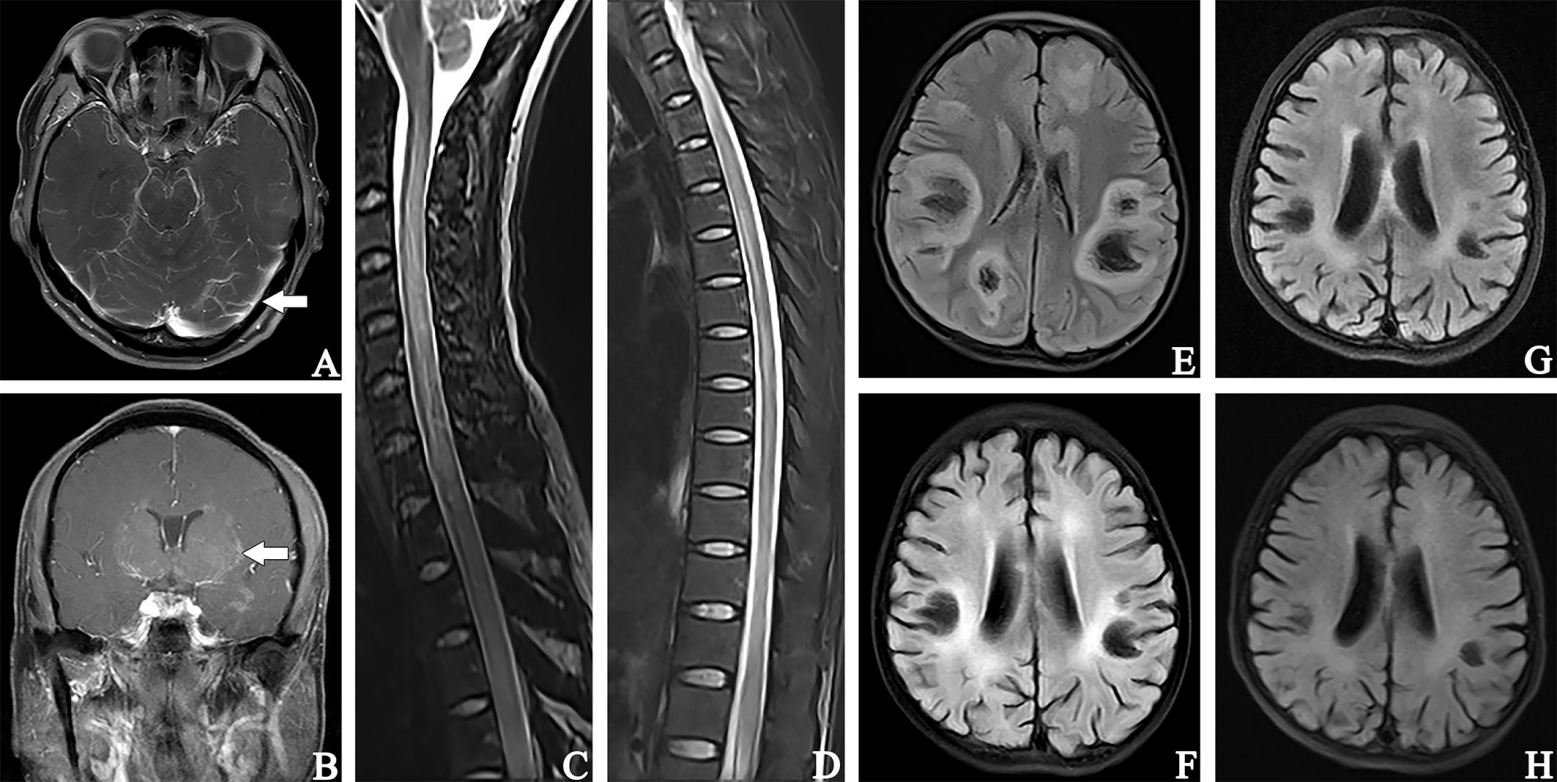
Contrast-enhanced T1-weighted magnetic resonance image (A, B). (A) patient #18, showed soft meningeal linear enhancement (arrow). (B) patient #23, MR image suggested patchy enhancement around the lateral ventricles (arrow). (C, D): patient #18, T2-hyperintense lesions in C2-T12. Fluid attenuated inversion recovery (E–H). MR images of patient #3. (E) MR image showed multiple lesions of bilateral brain parenchyma at admission. (F) (1 month after treatment with IVMP and IVIG) and (G) (2 months after treatment) showed that the extent of lesions gradually decreased and brain atrophy began to appear. (H) 4 months after treatment, a significant reduction in the lesion range and high-signal lesions in the bilateral lateral periventricular. The widening and deepening of the cerebral sulcus and fissure were evident.
